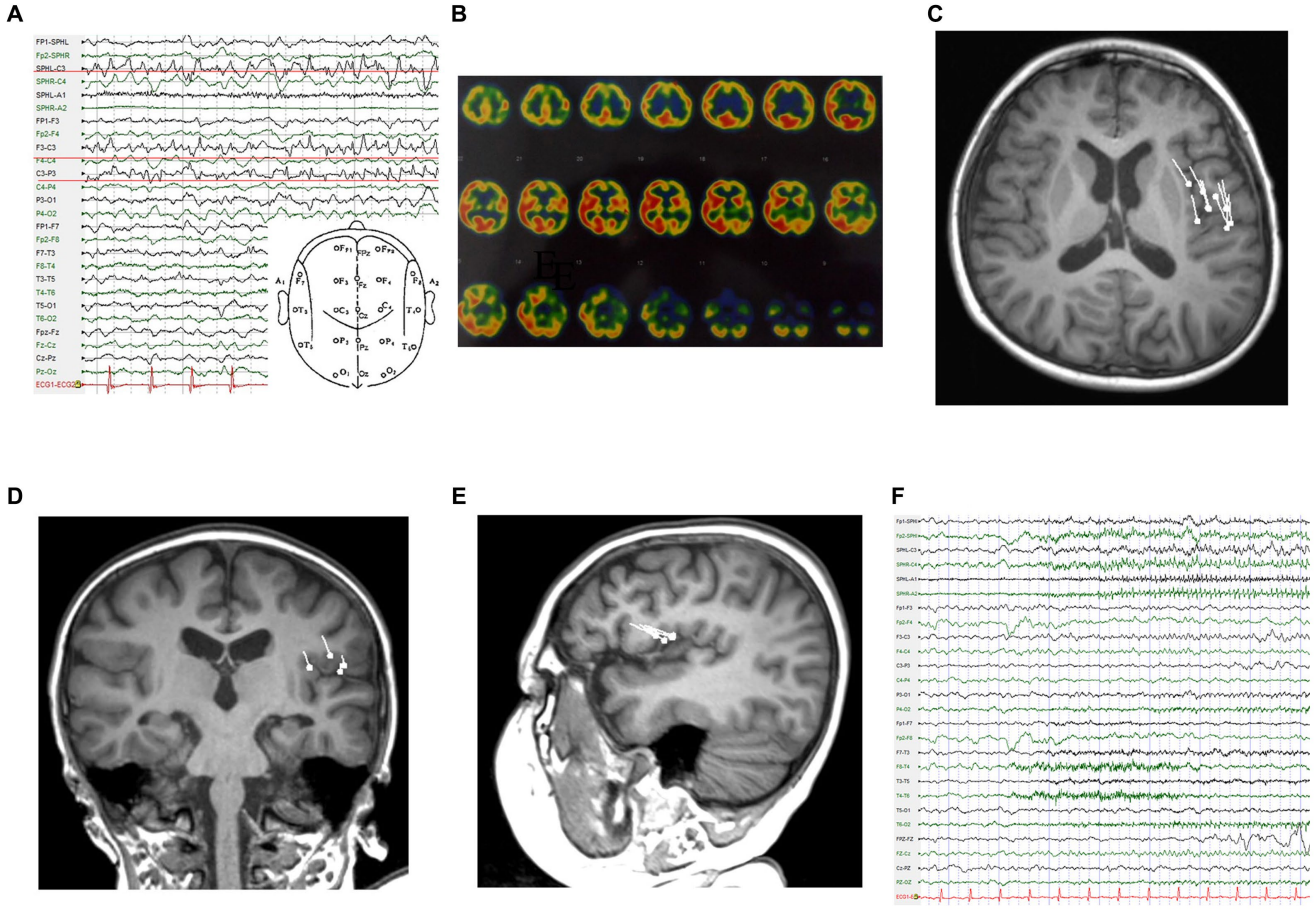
Examinations in the first admission. (A) Interictal EEG. (B) SPECT reveals markedly hypointense areas. (C-E) Dipoles are concentrated in the left operculum on magnetoencephalography (C: T1 axial, D: T1 coronal, E: T1 sagittal). (F) Ictal EEG.
